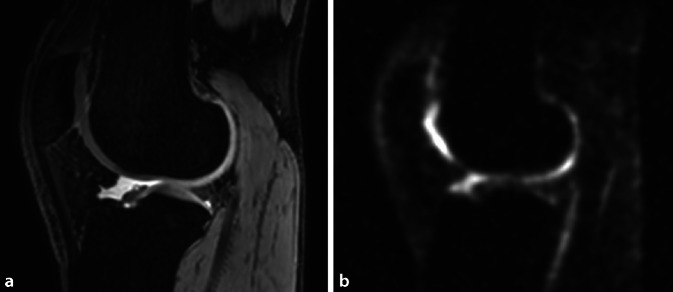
An image of the right knee of a 30-year-old male patient in a sagittal orientation generated with a proton (^1^H) MRI three-dimensional double-echo steady-state sequence (DESS) with an in-plane resolution of (0.5 mm)^2^ and, b sodium (^23^Na) three-dimensional density adapted radial projection (DA-3DPR) sequence with an isotropic resolution of (2.0 mm)^3^. The images demonstrate high morphological similarity; however, ^23^Na-MRI might provide additional information on the biochemical state of the cartilage tissue
